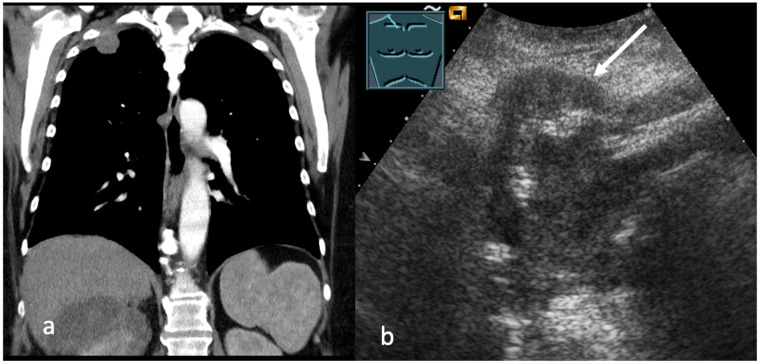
(a,b) Lung cancer in CT (a). B-US presents a tumor with complex echogenicity (b) and with infiltration of the thoracic wall (arrow).
